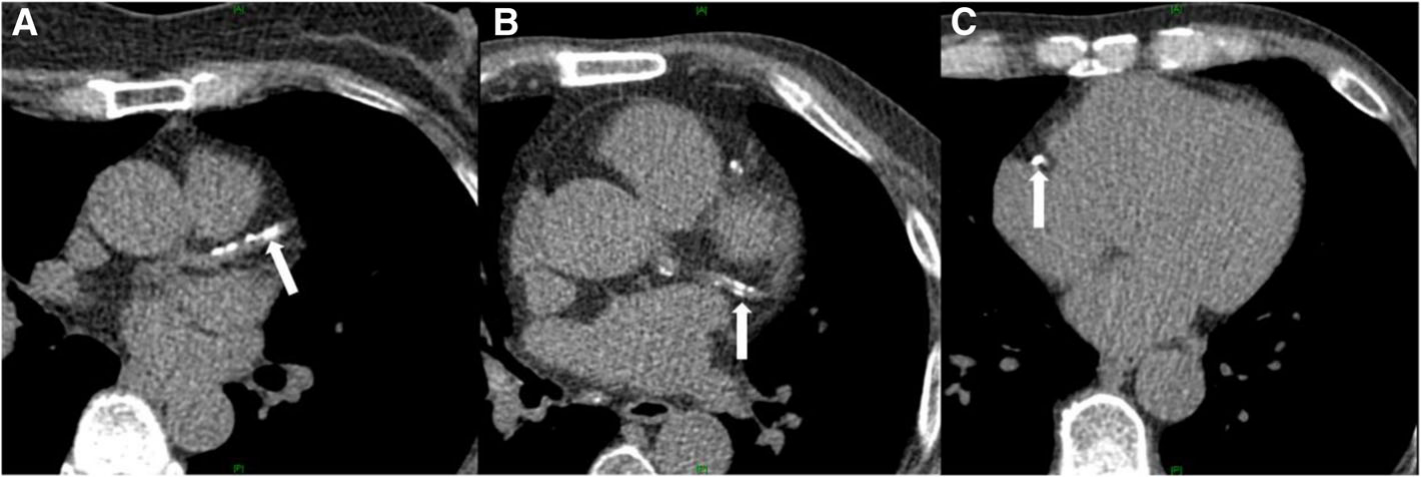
Computed tomography (CT) of coronary arteries. A representative calcium deposit image of coronary arteries detected in a CT scan of the heart. The white arrows indicate the calcium deposit in (a) left anterior descending, (b) left circumflex, and (c) right coronary artery
